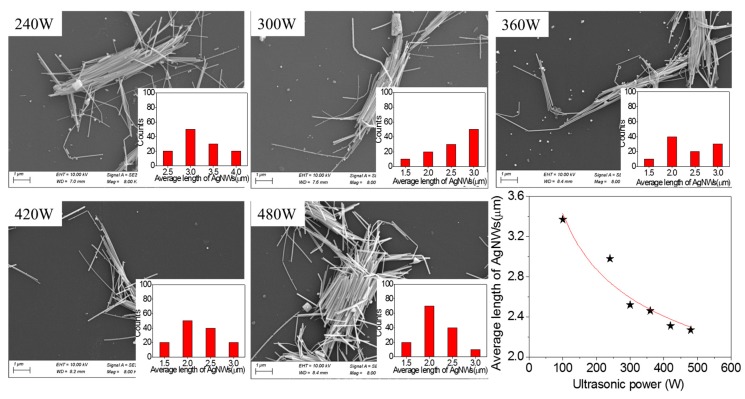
SEM images of AgNWs (1.67 mg·mL^−1^) sonicated for 3 h and different ultrasonic powers and the relationship of average length of AgNWs with ultrasonic power.
